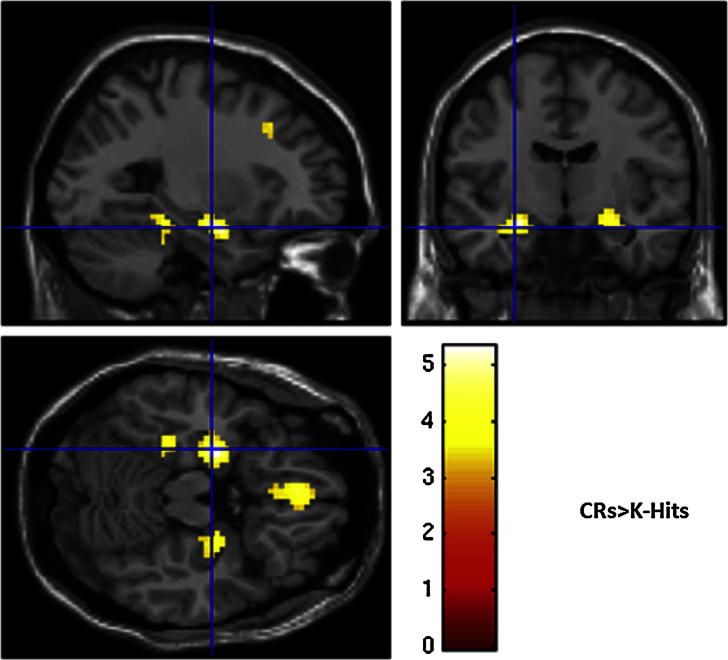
Sections through a canonical T1-weighted image in MNI space showing hippocampal regions that demonstrated greater BOLD signal for CRs than K Hits at *p* < .001 uncorrected for display purposes (maximum in left hippocampus survives *p* < .05 corrected for the whole-brain).
